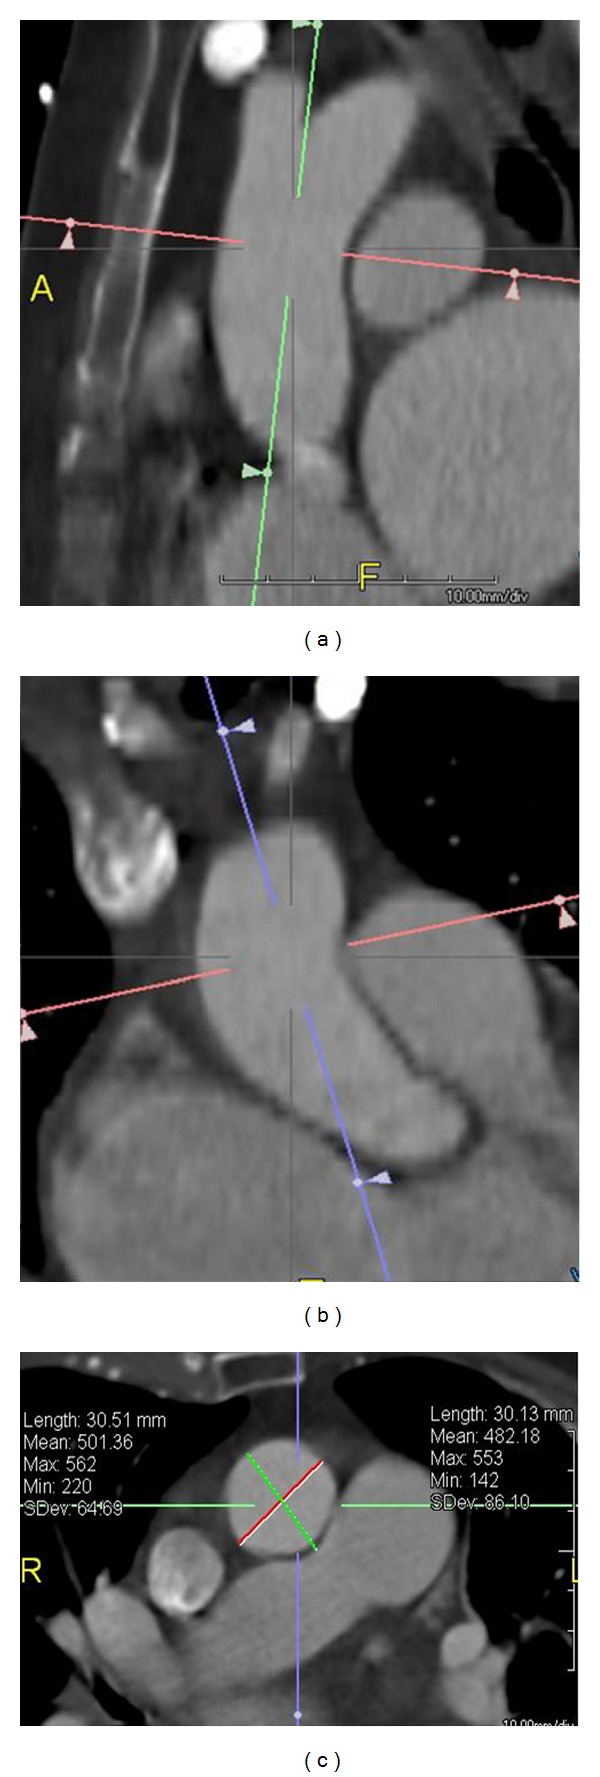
Measurement  of  aortic  size  by  CT  scan. Double oblique short axis image of mid ascending aorta (c) is acquired from orthogonal coronal (a) and sagittal (b) images at the level of mid ascending aorta. From the double oblique short axis image, minimum and maximum diameters and area are measured.
